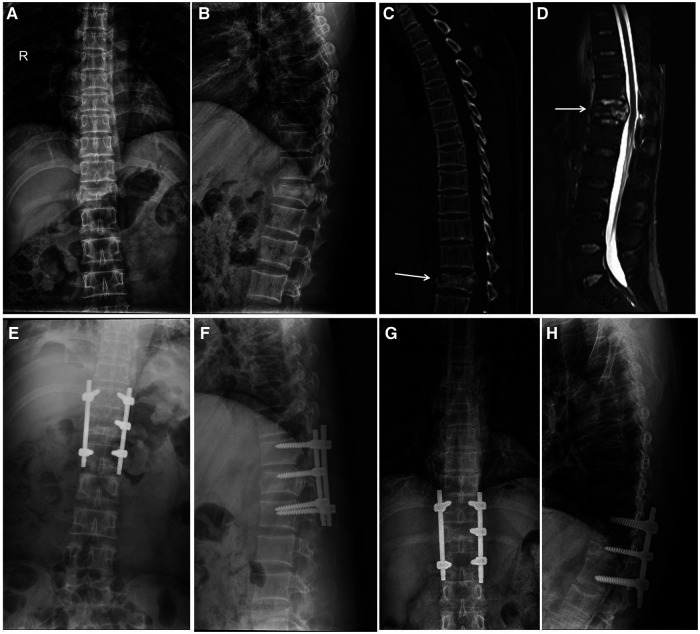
A 46-year-old male patient with T12 fracture and a decrease in anterior vertebral height >50% before surgery. (A–D) The preoperative x-ray, CT, and MRI images showed burst fracture of the T12 vertebra. (E,F) The patient underwent short-segment fixation including the fractured vertebra without transpedicular bone grafting. Immediate postoperative x-ray examination indicated that the height of T12 vertebral body was recovered. (G,H) x-ray examination, performed 2 year later, showed that the fracture vertebral body had collapsed and the height of the T12 vertebral body was decreased to 55.6%.
